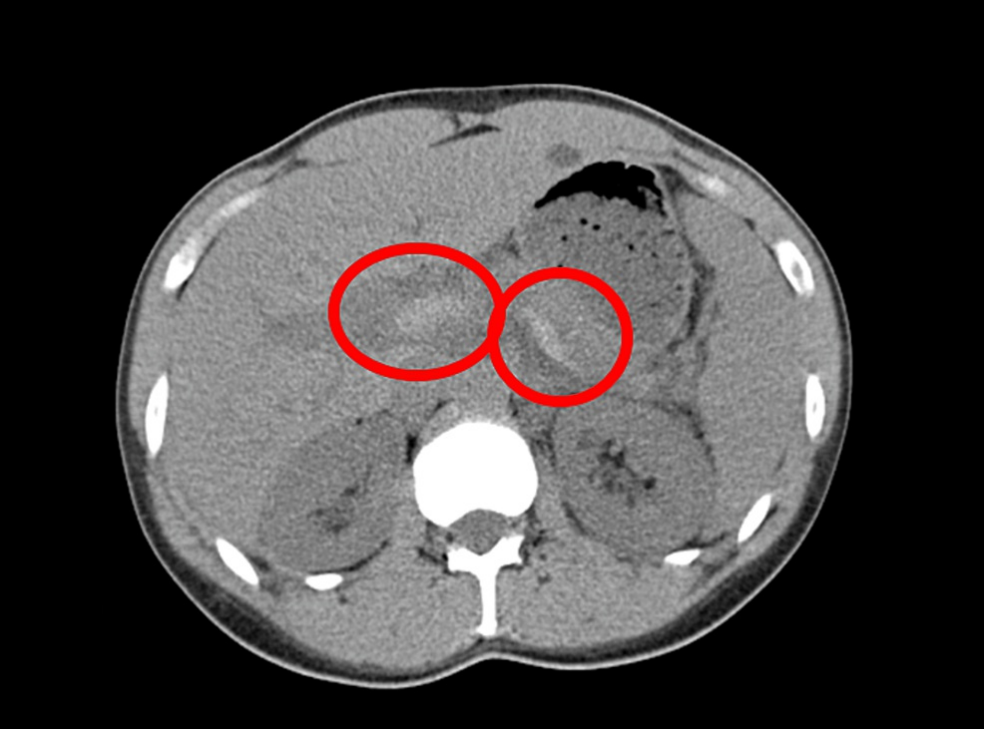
CT scan of the abdomen showing splenic and portal vein thrombosis (circle) CT: computed tomography
